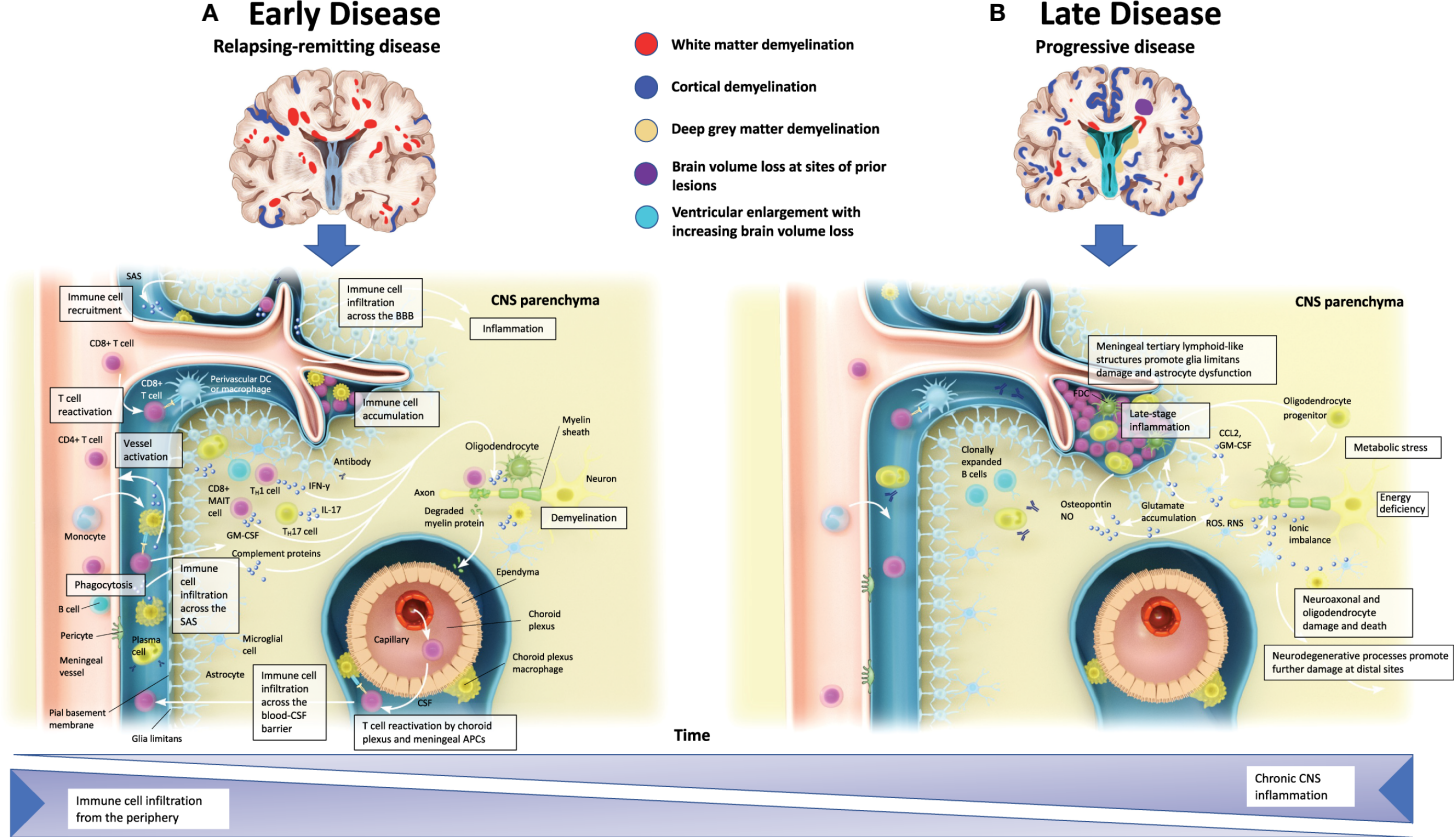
Pathophysiology of multiple sclerosis. (A) In the early stages of MS, relapses coincide CNS inflammation and demyelination that are typically discernible as white matter lesions via MRI. Peripheral immune cell infiltration can occur from meningeal blood vessels (across the BBB), the subarachnoid space, or the choroid plexus (across the blood-CSF barrier). These infiltrates may then accumulate in perivascular spaces and enter the CNS parenchyma, and along with activated CNS-resident microglia and astrocytes, promote demyelination and oligodendrocyte and neuroaxonal injury through direct contact-dependent mechanisms or via soluble inflammatory and neurotoxic mediators. (B) In the later stages of MS, progressive neurological decline is accompanied by CNS atrophy. Immune cell infiltration is dampened, but CNS inflammation persists. Meningeal tertiary lymphoid-like structures can also contribute to late-stage inflammation in SPMS. Irrespective of MS subtype, CNS-resident innate immune cells may contribute to chronic inflammation. Astrocytes produce ligands and factors that can promote microglial recruitment and activation while also preventing remyelination at the sites of neuroaxonal injury by inhibiting progenitor cell development into mature oligodendrocytes. APC, antigen-presenting cell; BBB, blood-brain barrier; CCL, chemokine ligand; CNS, central nervous system; CSF, cerebrospinal fluid; FDC, follicular dendritic cell; GM, grey matter; IFN-y, interferon gamma; IL, interleukin; MAIT, mucosal-associated invariant T cell; MRI, magnetic resonance imaging; MS, multiple sclerosis; NO, nitric oxide; RNS, reactive nitrogen species; ROS, reactive oxygen species; SPMS, secondary progressive multiple sclerosis; TH, T helper. Adapted from Dendrou et al. (38).
